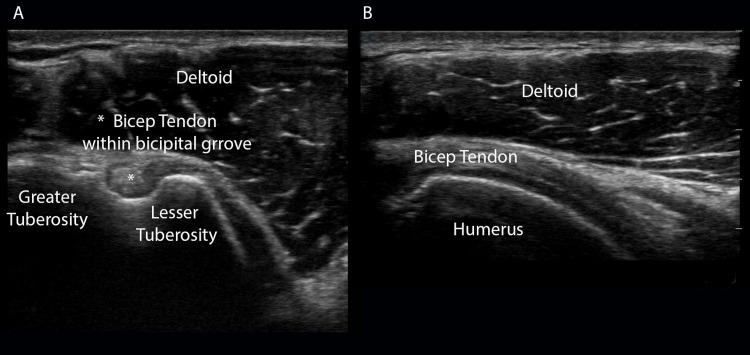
Long Head of the Biceps Tendon (A) Long head of the biceps tendon in transverse as it travels through the bicipital groove between greater and lesser tuberosities and (B) 90-degree clockwise rotation of the ultrasound probe will reveal a long axis view of the bicep tendon.
